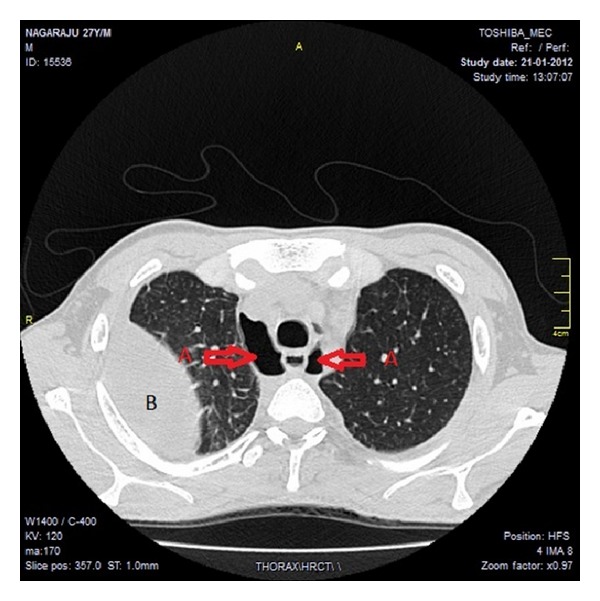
HRCT thorax (a) A indicating air in the mediastinum, (b) B indicating collection of pus in the pleural cavity.
